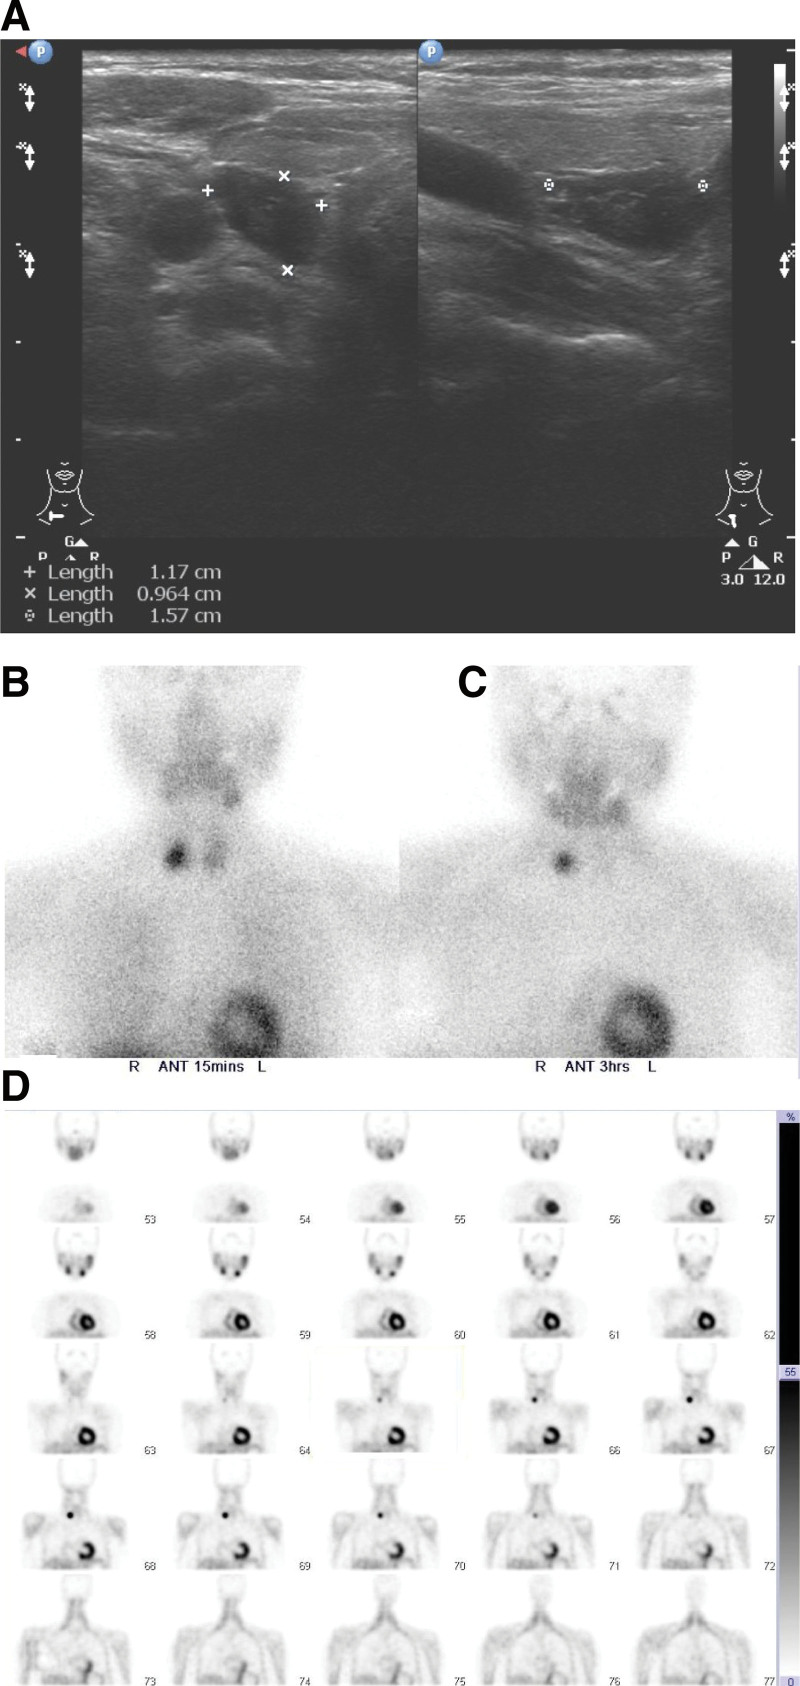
Cervical ultrasonography showing a heterogeneous hypoechoic well-defined mass in the right thyroid bed measuring 1.17 × 0.96 × 1.57 cm in maximal dimension. (A); Tc-99m MIBI showing a focal hot spot of tracer accumulation at the right lower thyroid bed at 15 minutes and (B); at 3 hours delayed phase (C); SPECT did not show definite abnormal foci of tracer activity in the thorax (D). Tc-99m MIBI = Technetium-99m methoxy-isobutyl-isonitrile scintigraphy, SPECT = Sestamibi-single photon emission computed tomography.
